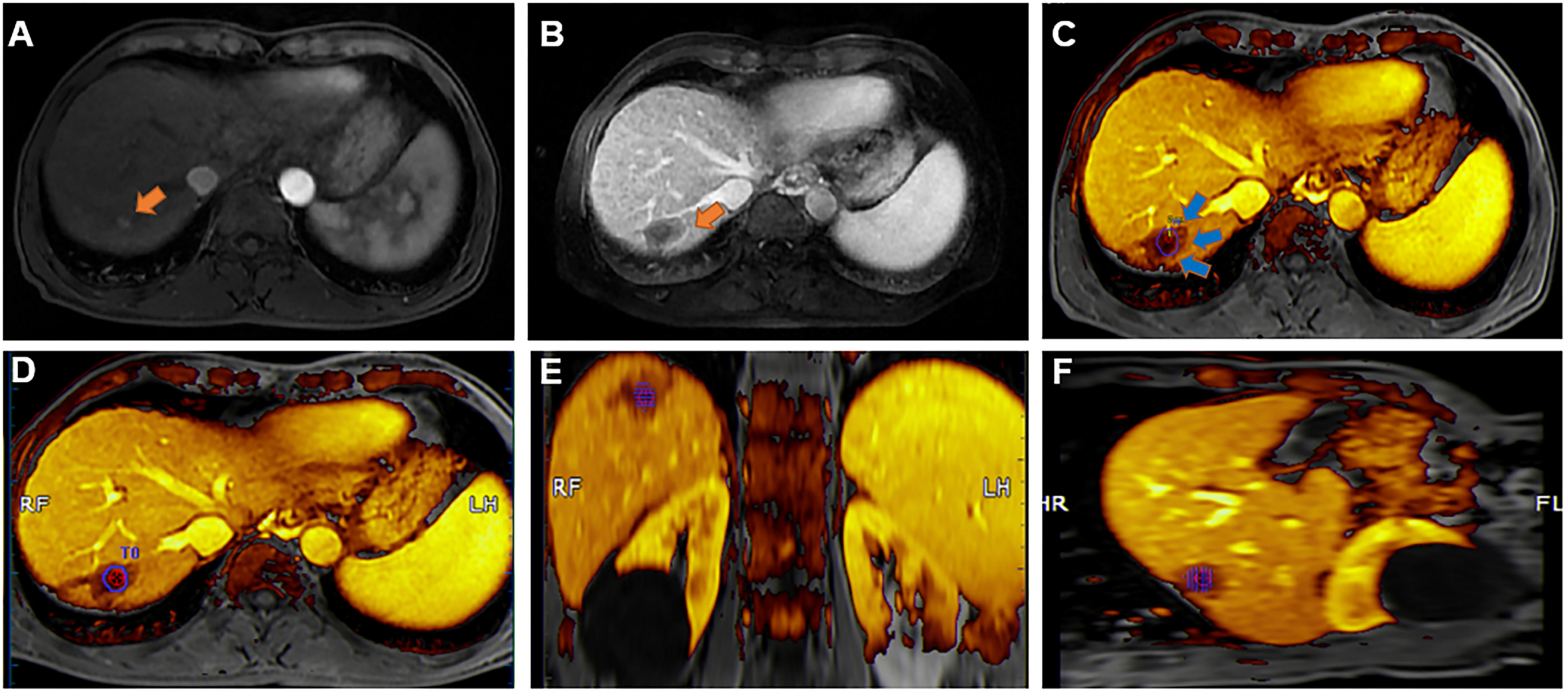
An example of margin assessment based on MR-MR fusion. (A) Co-registered pre-ablation MR images show the tumor zone (orange arrow); (B) Co-registered post-ablation MR images show the ablation zone (orange arrow). (C) Achievement of registration between the tumor and ablation zones (blue arrow). (D–F). Segmented tumor (red) and theoretical 5 mm (blue) margin contours overlaid on the ablation zone on the axial, sagittal, and coronal MR images.
